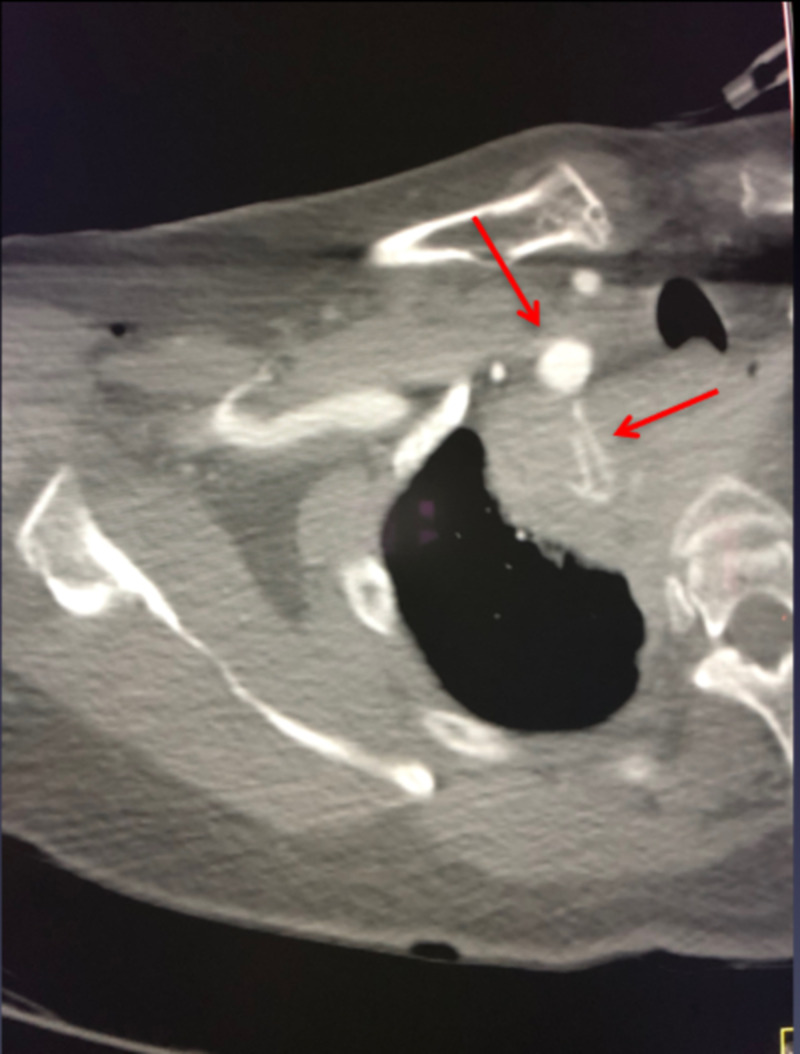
Axial CT angiogram of the chest showing active contrast extravasation from the right subclavian artery.
